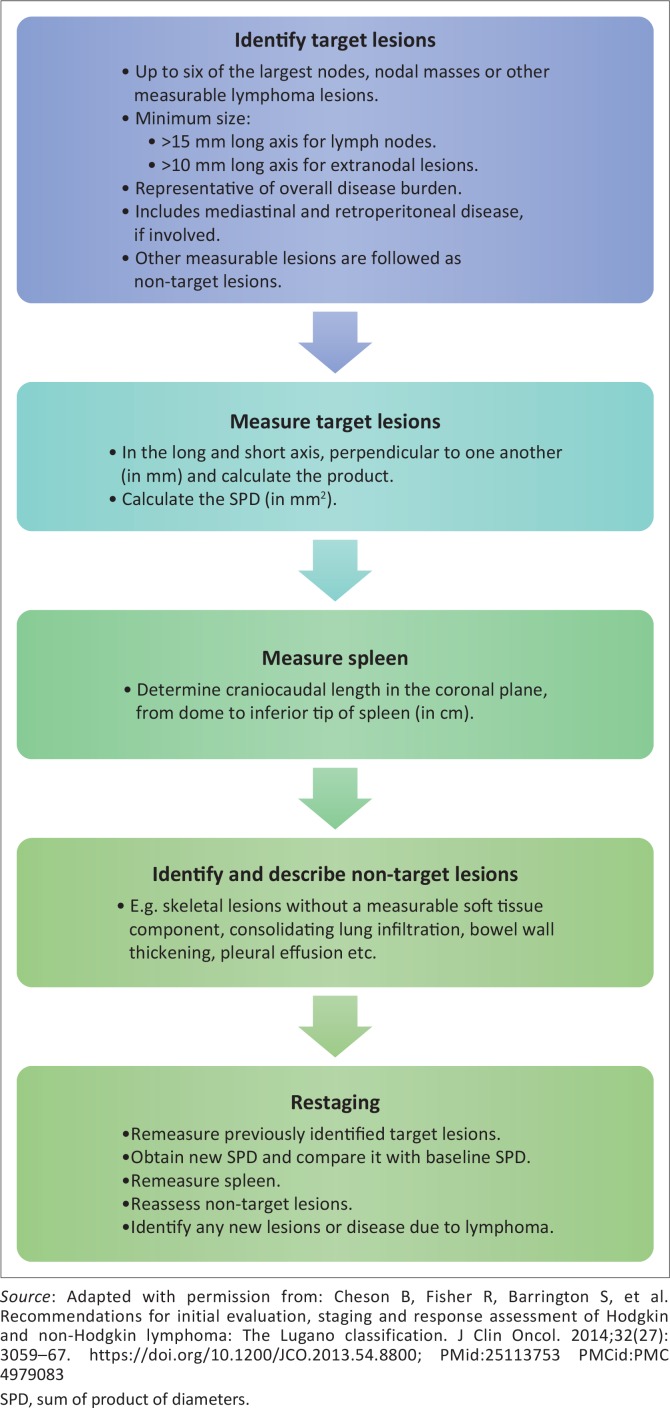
Applying the Lugano classification with computed tomography.
